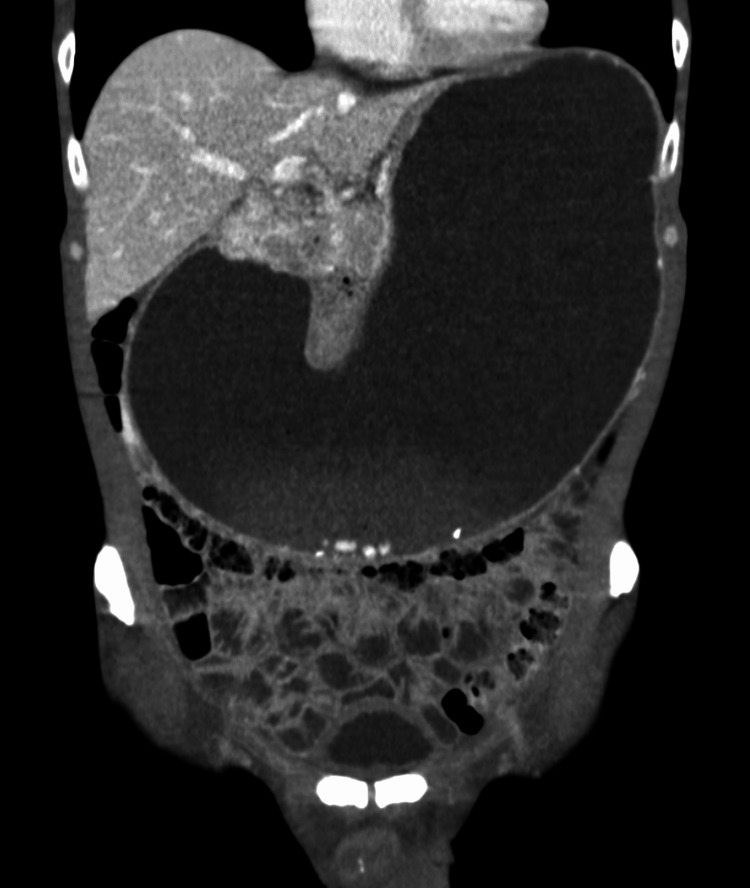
Computed tomography of the abdomen. The figure shows a coronal section of the abdominal computed tomography with intravenous contrast enhancement in the arterial phase. The stomach occupies the upper two-thirds of the abdomen and compromises the abdominal organs, including the intestinal loops in the lower abdomen and the liver in the upper left of the image. Note also the compromised colon throughout its course. A suspected heterogenous mass can be recognized in the pyloric region. The liver is free of metastasis.
